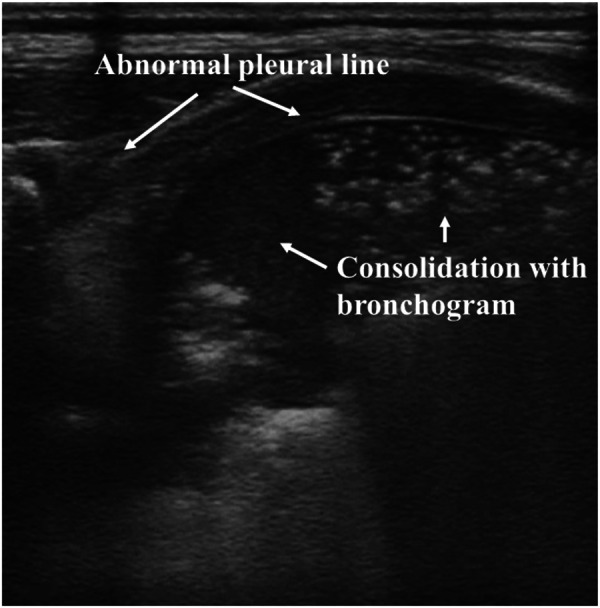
Atelectasis on lung ultrasonography. Ultrasound shows abnormal pleural lines, absent A-lines, and large areas of consolidation.
